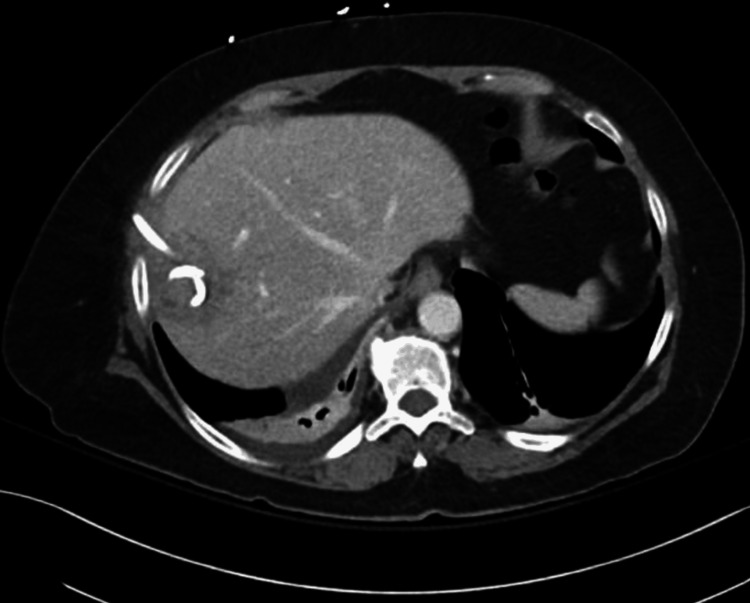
Post-procedural CT abdomen revealed the placement of a surgical drain within the liver abscess and its drainage. The white opaque object seen in the right lobe of the liver at the lesion site suggests the surgical drain.
